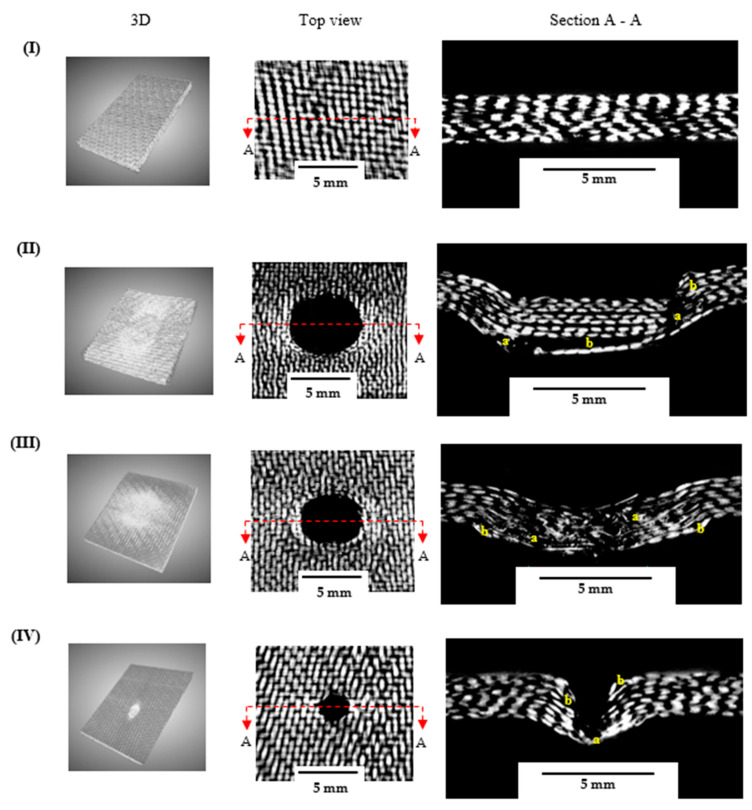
Post mortem μ-CT analysis for GL samples: (I) undamaged, (II) GLS, (III) GLH, (IV) GLC. Note: a—fibre breakage, b—delamination.
